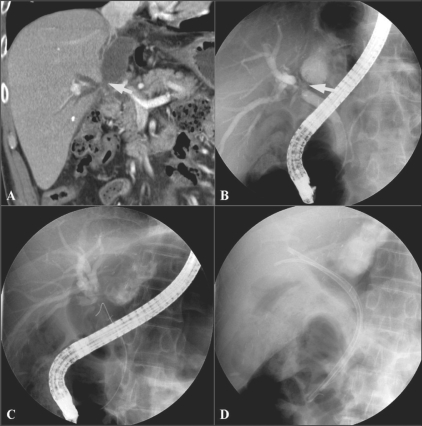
A 52-year-old man who had undergone a liver transplantation presented with fever. Coronal MPR CT scan (A) shows dilated IHDs and a loculated fluid collection near the CHD (arrow). Bile leak at the anastomotic site of the CHD and communication between the biloma and CHD was suspected. ERCP (B) in the same plane, reveals bile leak at the anastomotic site (arrow). Endoscopic CBD stent insertions (C, D) were performed. MPR: multiplanar reconstruction, CHD: common hepatic duct, IHD; intrahepatic duct
